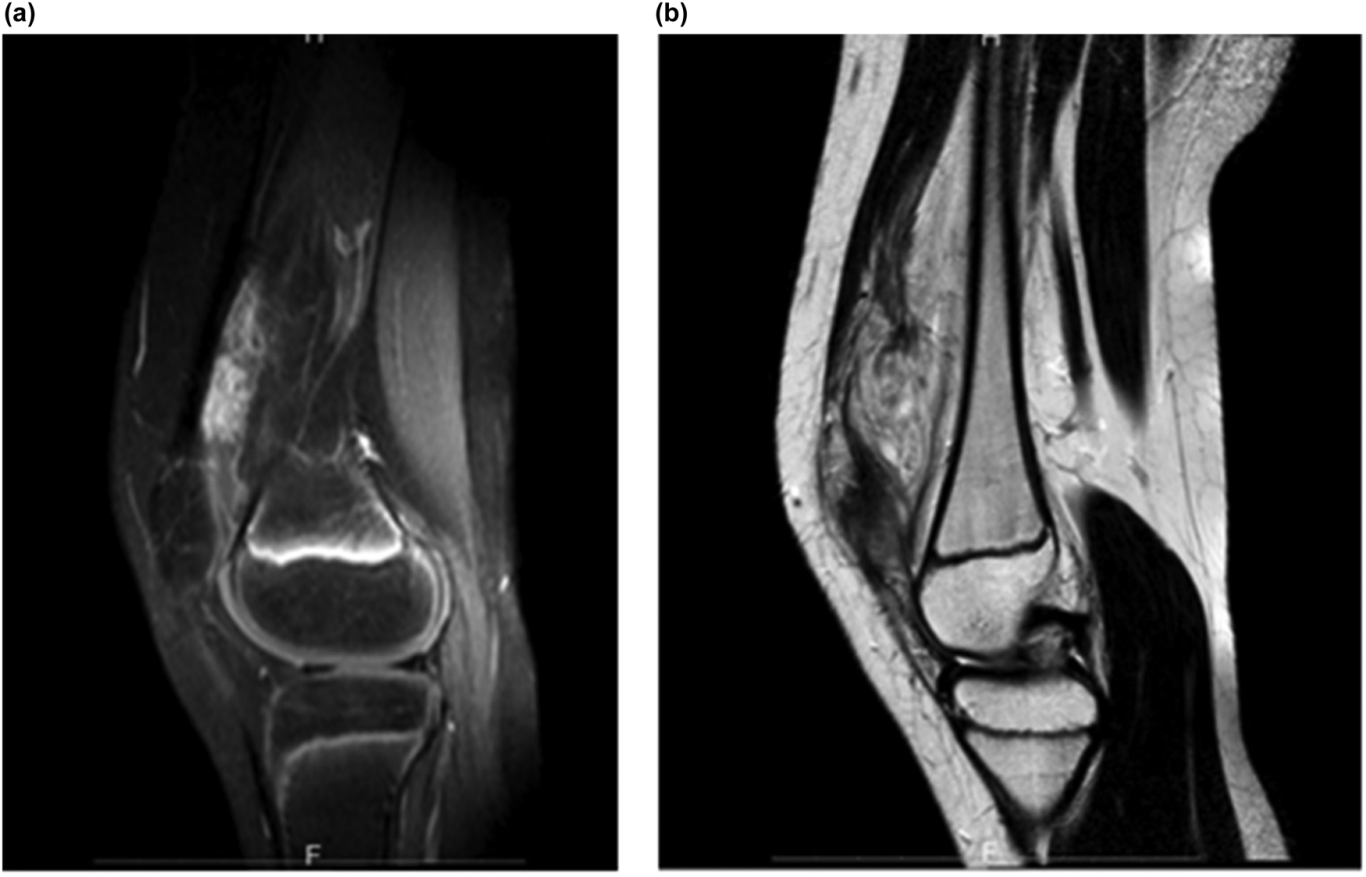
Follow-up magnetic resonance imaging at 3 years postoperative. (a and b) Both images show the minimal fibroadipose gaps in the vastus medialis muscle.
